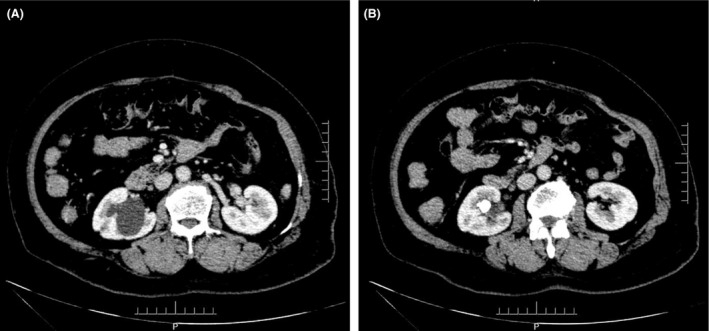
(A and B) Preoperation CT.
